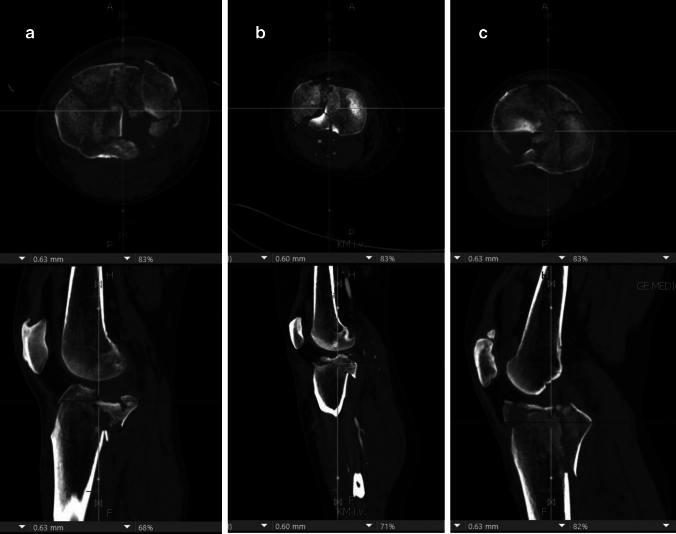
CT (axial and sagittal, coronal not shown) of the three cases. a case 1, b case 2, c case 3
